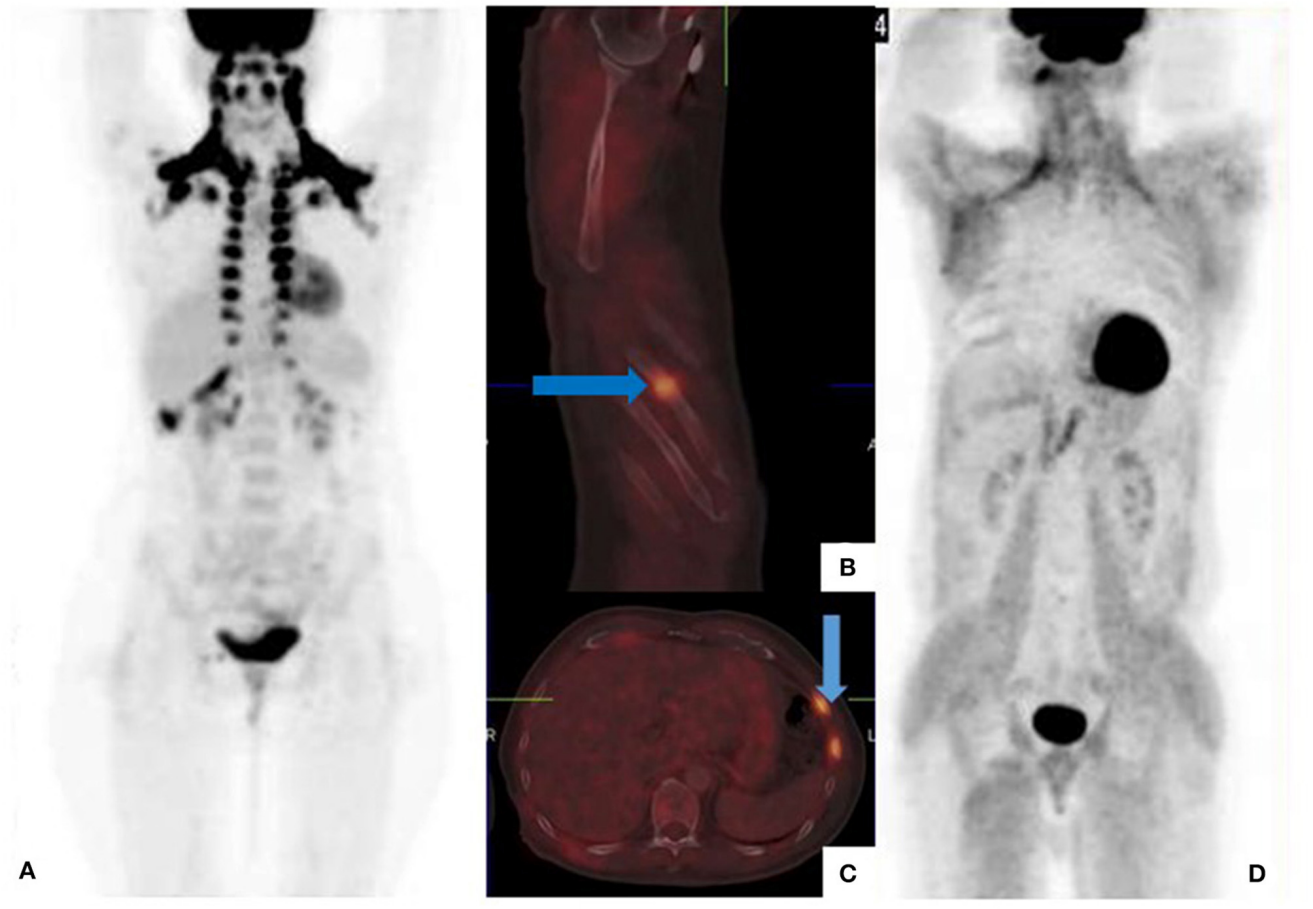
[18F]FDG PET/CT for staging malignant melanoma of the upper lip (A) and back (B–D). Maximum intensity projection (MIP) shows symmetrical brown fat uptake in the cervical, supraclavicular, mediastinal, and paraspinal regions (A). Fused sagittal (B) and trans-axial (C) images show increased tracer uptake in the 6th, 7th, and 8th anterior-lateral consecutive ribs with callus formation noted on CT. These findings represent fractures from previous trauma and not metastatic disease. Generalized muscle uptake (D) was noted in a patient who inadvertently injected his insulin on the morning of the scan.
